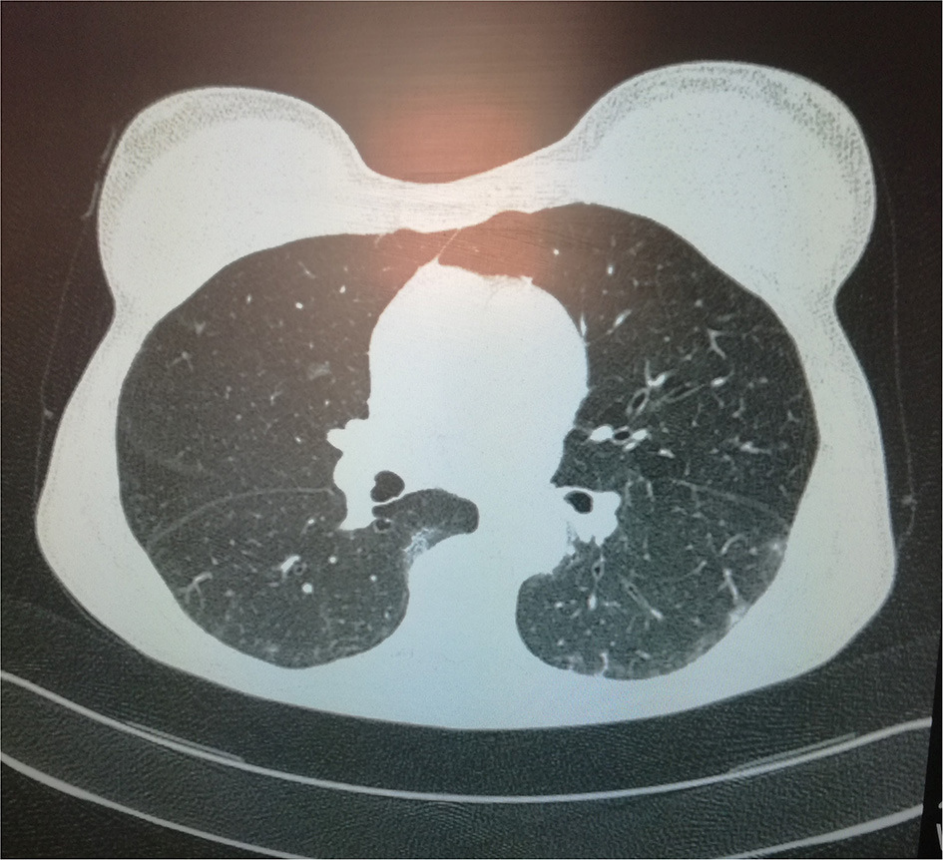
A chest computed tomographic image in the axial plane demonstrates ground-glass opacities of the lung parenchyma with bilateral and sub-pleural distribution admixed with areas of focal consolidation.
